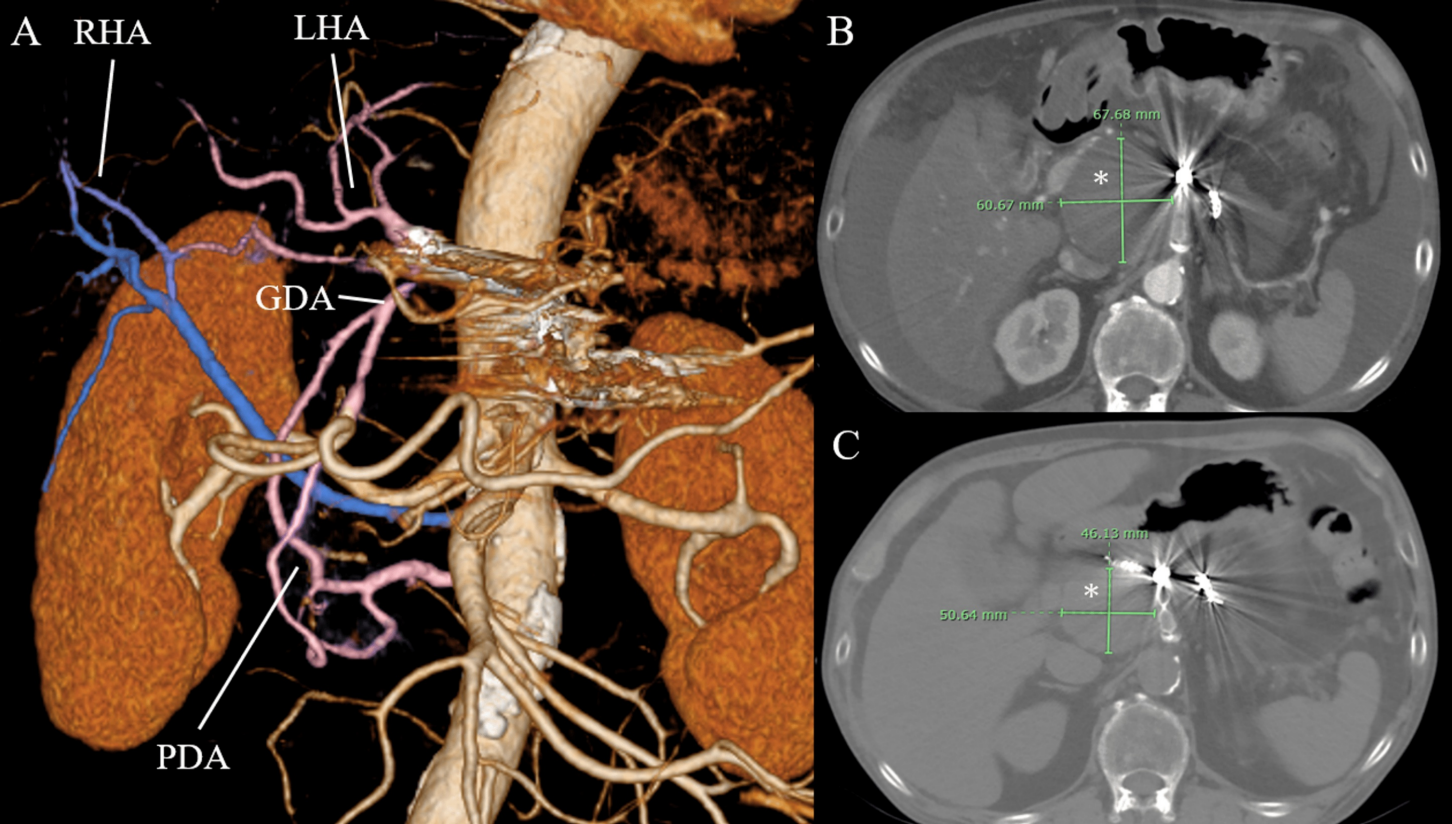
Postoperative CT findings. Asterisks indicate celiac artery aneurysm. (A) CT image 1 month after surgery; (B) Diameter of the celiac artery aneurysm 1 month after surgery; (C) Diameter of celiac artery aneurysm 1 year after surgery. RHA: right hepatic artery; LHA: left hepatic artery; GDA: gastroduodenal artery; PDA: pancreaticoduodenal arcade.
